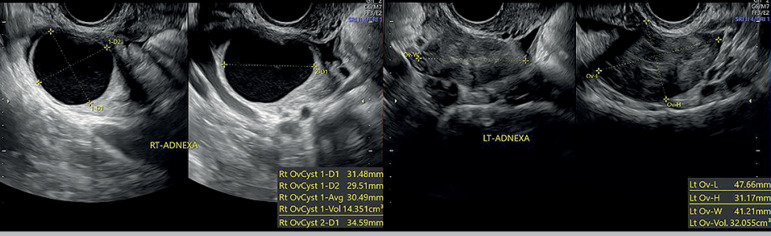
Pelvic ultrasound performed during the follicular phase three months after surgery.
